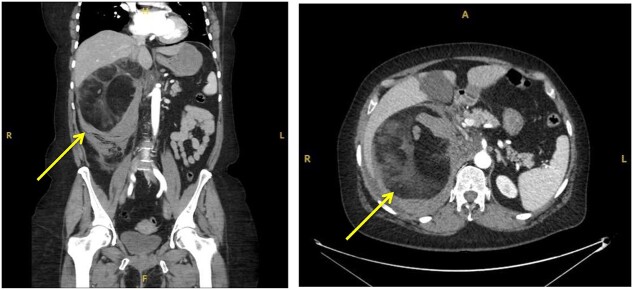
Coronal (a) and transverse (b) sections of initial CT imaging with contrast showing large lesion with heterogeneous hypodense areas with surrounding haematoma (arrowed).
